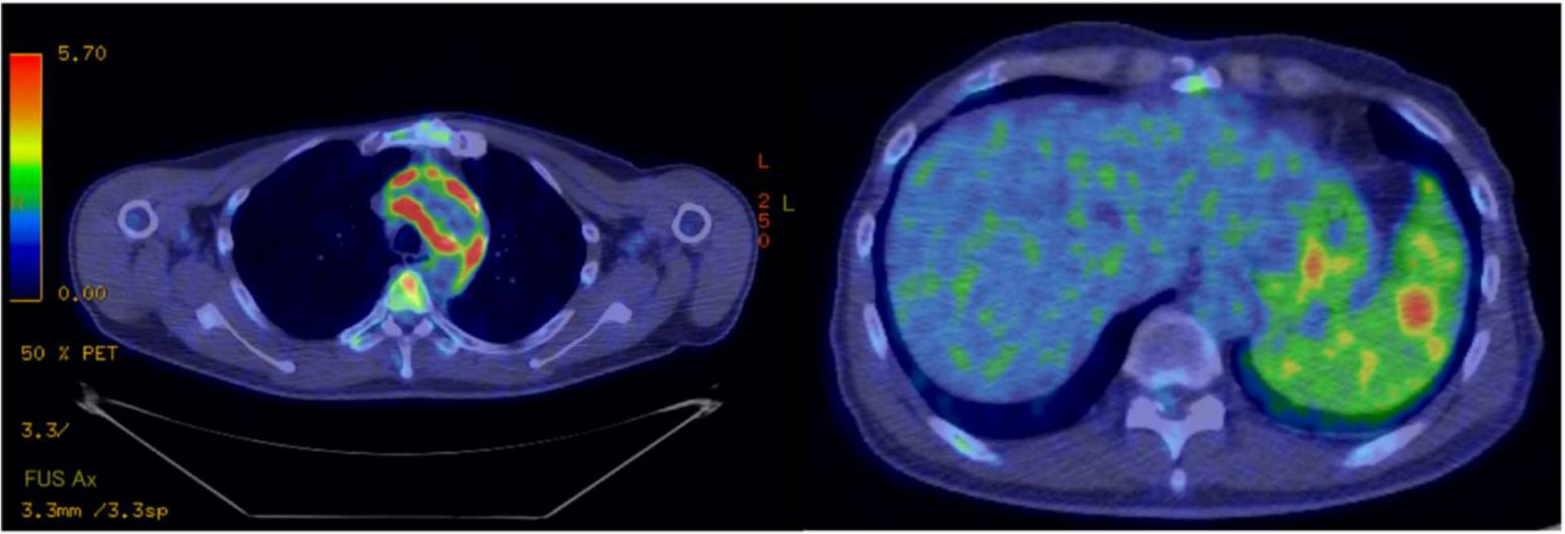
Positron-emission tomography-computed tomography. Hyperaccumulation (SUVmax: 11.8) in the fluid collection around the graft (left). Hyperaccumulation was observed throughout the spleen (SUVmax: 4.1), and localized hyperaccumulation was consistent with the area of splenic infarction (SUVmax:5.5) (right)
